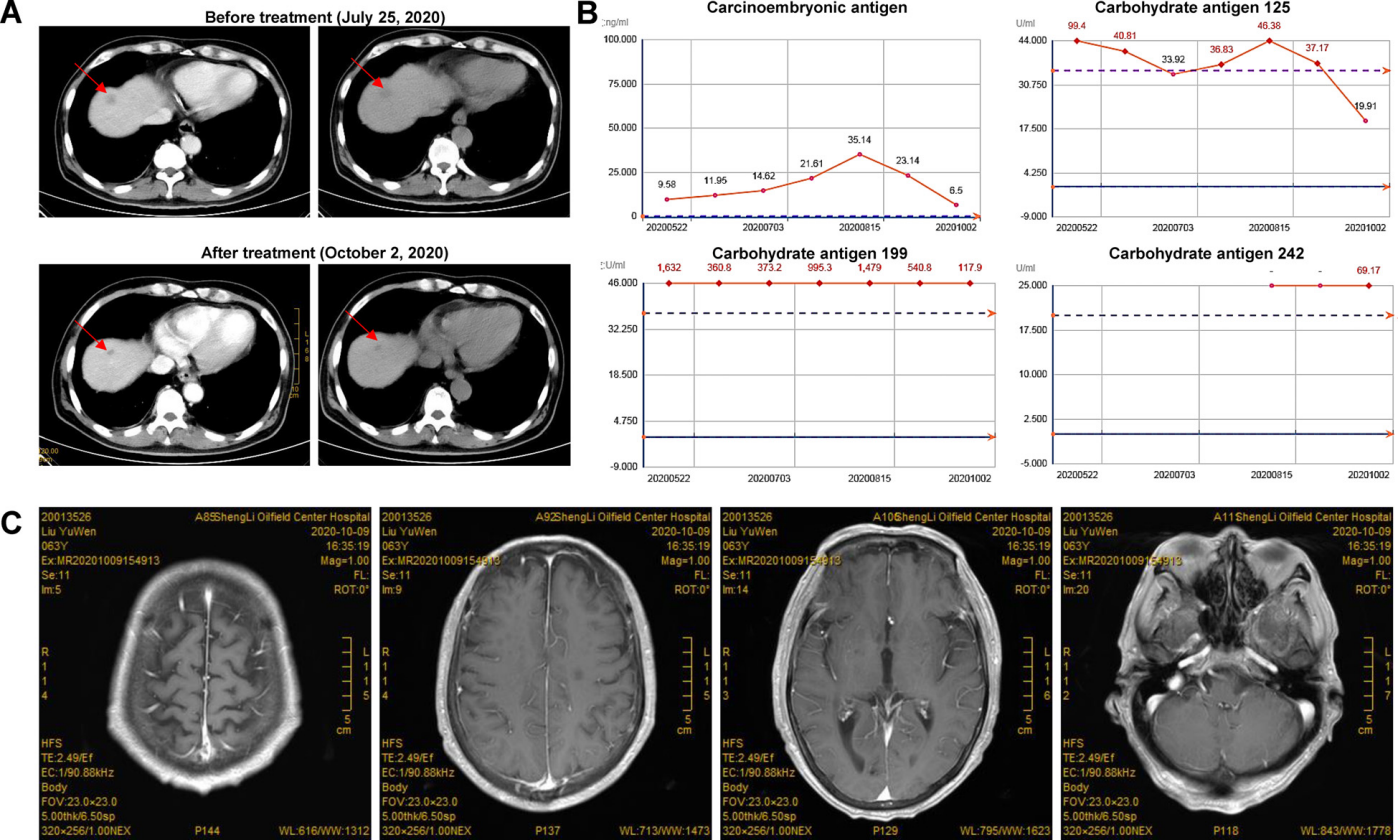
Tumor and biomarkers change in a patient with dermatomyositis after sintilimab plus nab-paclitaxel. (A) The abdominal CT indicated a reduction in liver masses after treatment; (B) The levels of tumor markers were decreased after treatment. Carcinoembryonic antigen, carbohydrate antigen (CA) 125, CA 199, and CA 242 were detected with electrochemiluminescence assay using commercial kits (Roche Diagnostics GmbH, Penzberg, Germany). (C) Enhanced brain MRI revealed the old cerebral infarction and white matter ischemic changes, excluding the central nervous system metastasis.
